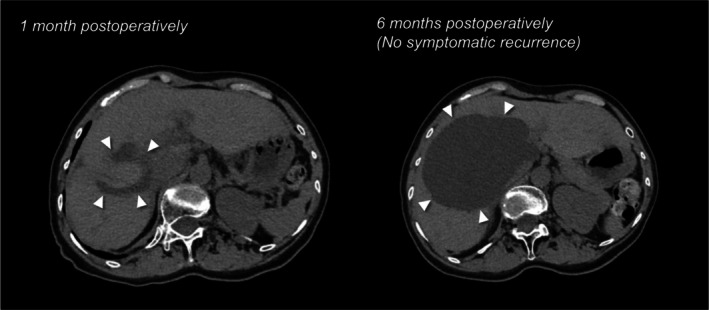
Postoperative CT findings in the present case. Postoperative CT at 1 month (left image) demonstrated marked reduction of the hepatic cyst (arrowheads). At 6 months postoperatively (right image), radiologic enlargement was observed (arrowheads), while the cyst remained smaller than its preoperative size and no recurrence with symptoms was noted.
